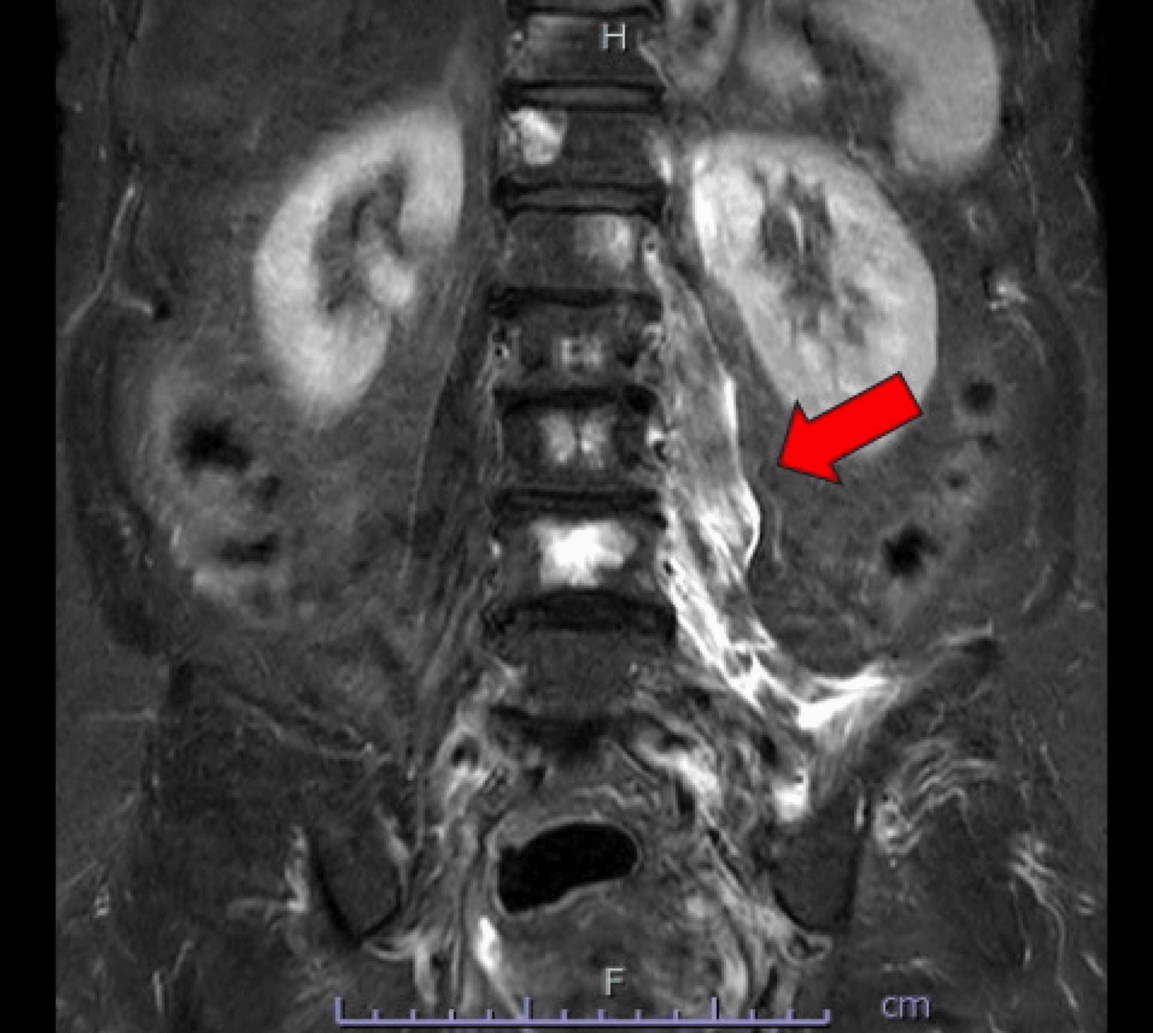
Magnetic resonance imaging findings. High signal intensity in the left psoas major and iliac muscles on short tau inversion recovery (arrow); no evidence of neurological involvement.
